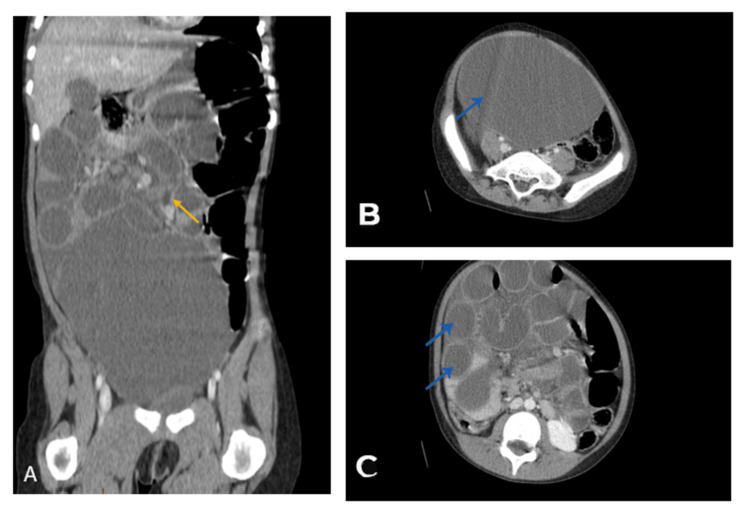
CT scan images. A: Coronal section of an abdominopelvic CT scan in the portal phase showing loops of the bowel (yellow arrow). B. Axial section of an abdominopelvic CT scan in the portal phase demonstrating an abdominopelvic mass consistent with cystic lymphangioma (blue arrow). C. Axial section of an abdominopelvic CT scan in the portal phase showing distension of intestinal loops (blue arrow).
